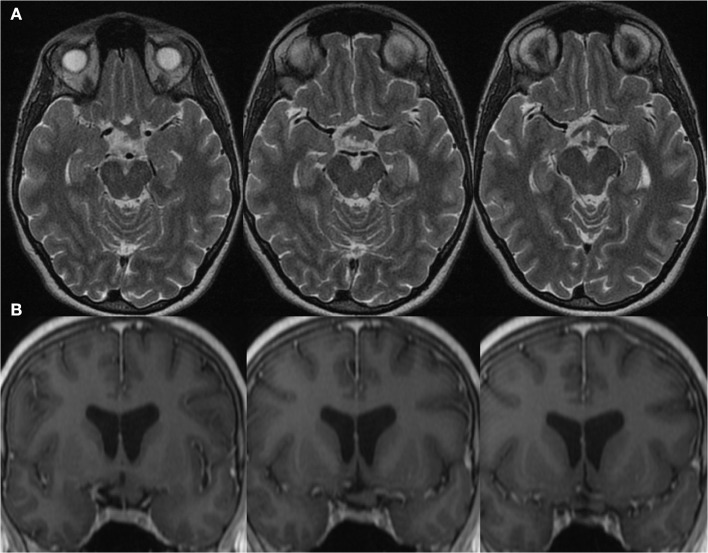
Magnetic Resonance Imaging of a left retro-chiasmal optic pathway glioma visualized on (A) Axial T2-MRI and (B) Coronal T1 post gadolinium MRI.
